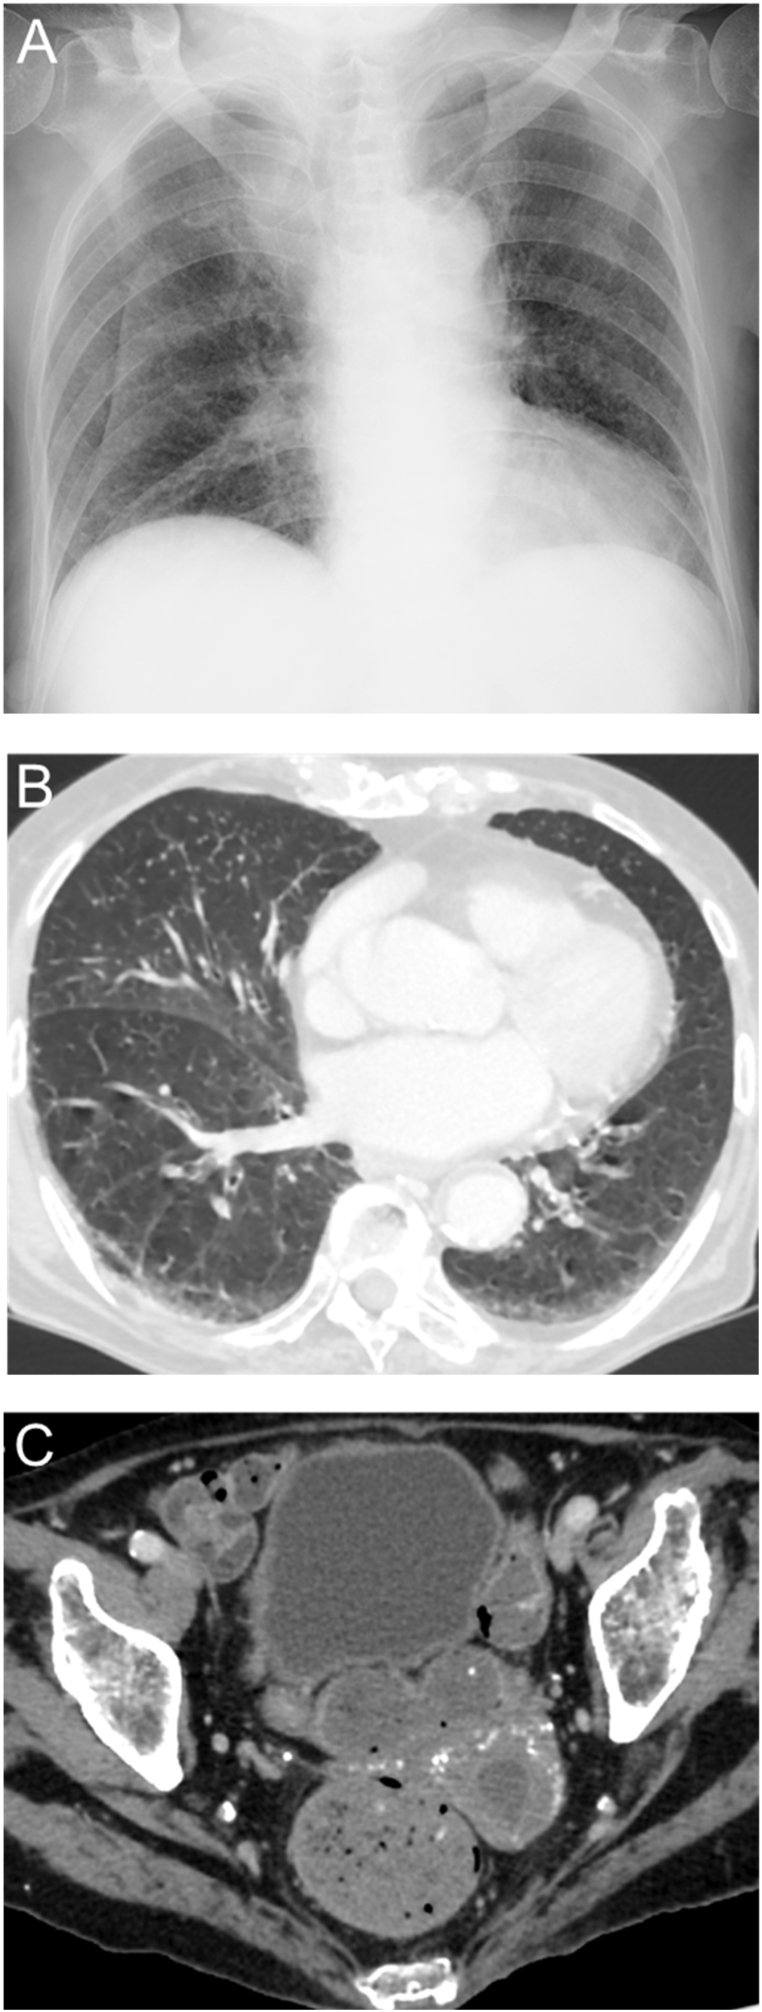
CT findings at one month after anti-tuberculosis treatment. A. Chest CT demonstrated an improvement in the diffuse, bilateral, granular opacities. B. Abdominal and pelvic CT demonstrated a reduction in the size of the pyometra.
